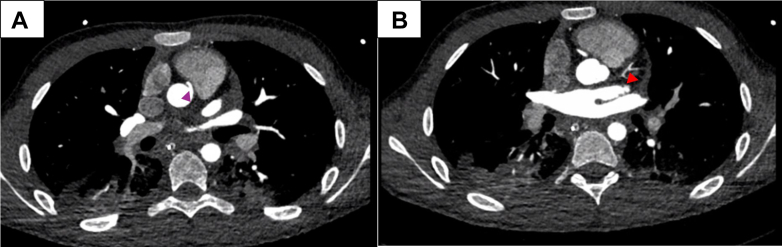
Initial Computed Tomography Scan Computed tomography scan (A) demonstrating the anomalous origin of the right coronary artery from the left facing coronary sinus, with an intra-arterial course between the aorta and pulmonary artery (purple arrowhead). (B) Left main coronary artery was suspected to be congenitally aplastic, with a hypoplastic left anterior descending coronary artery (red arrowhead).
